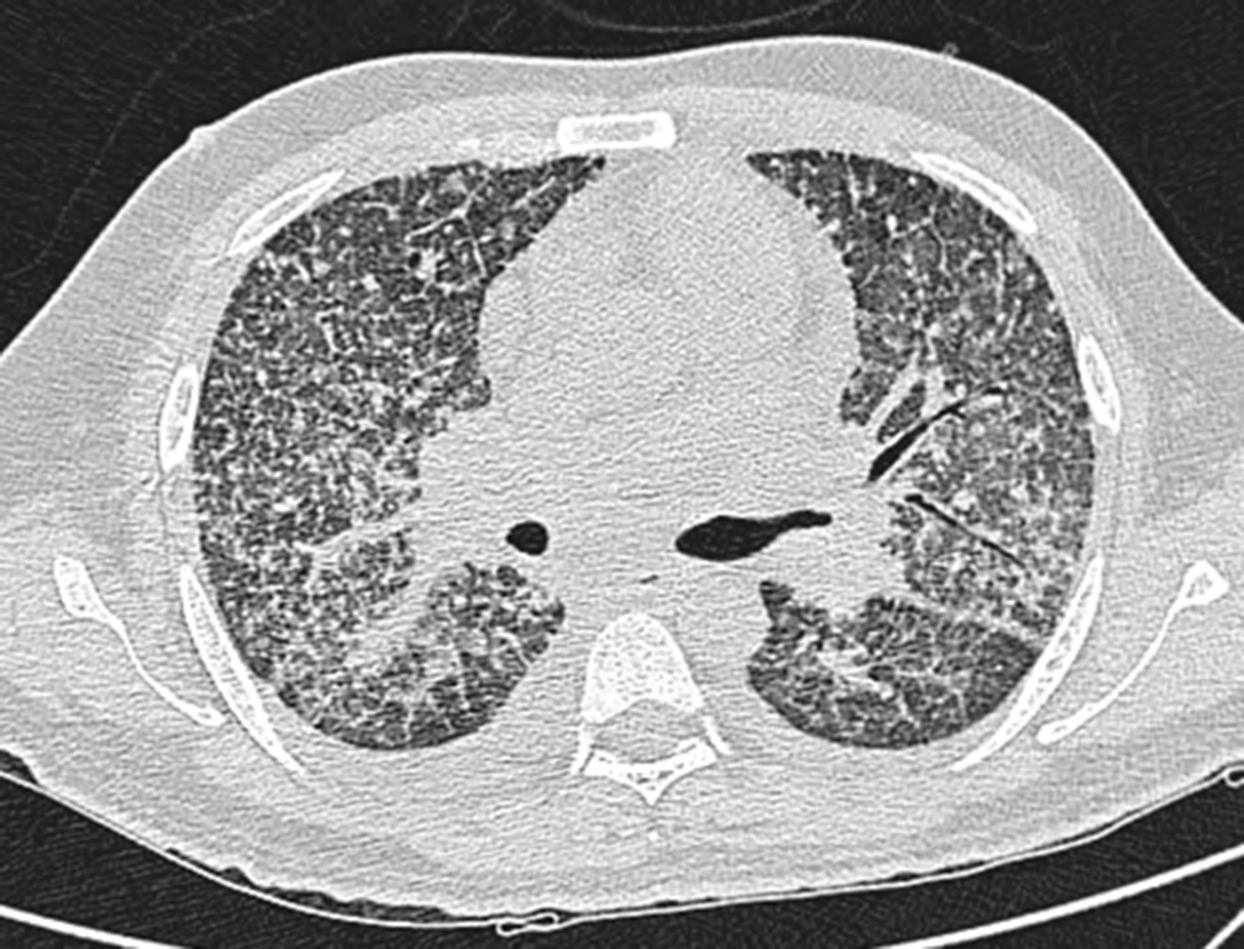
Case 1(b): CT scan of the chest in lung window shows multiple nodular opacities distributed along the peribronchovascular interstitium and subpleural regions (perilymphatic).
